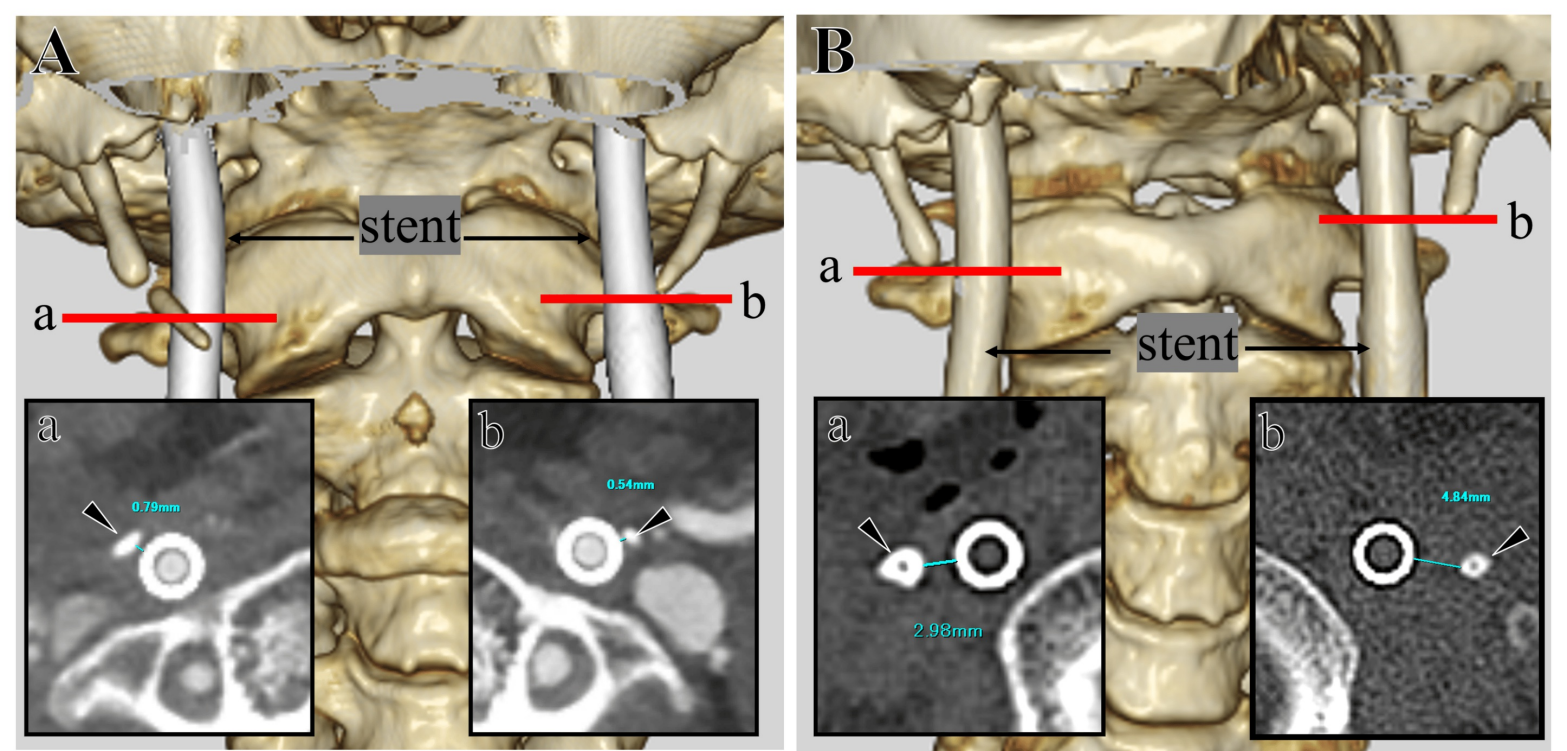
Three-dimensional (3D) reconstructed and axial CT imaging before and after bilateral styloidectomies. Preoperative 3D image showing the close proximity of the bilateral SPs to their respective ICAs (A). Preoperative axial images at the levels indicated by red lines demonstrate a close relation between the SPs (arrowhead) and stent-loaded ICAs, measuring 0.8 mm on the right (A-a) and 0.5 mm on the left (A-b). Postoperative 3D image after partial resection of both SPs (B). Postoperative axial images at the levels indicated by red lines demonstrating increased separation between the SPs (arrowhead) and stent-loaded ICAs, measuring 3.0 mm on the right (B-a) and 4.8 mm on the left (B-b). ICA: internal carotid artery; SP: styloid process
